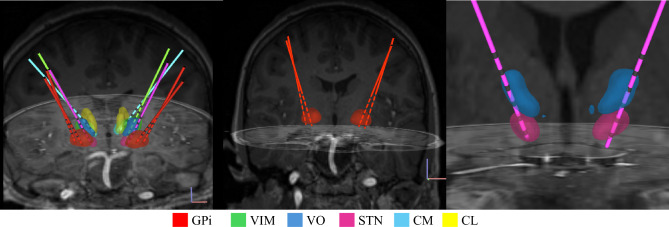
Postoperative localization of implanted DBS leads based on co-registered MRI and CT images for subject S13. The left panel displays all implanted leads and their target regions. For this subject, five leads were implanted in each hemisphere: two in GPi (red), one in VIM (green), one passing through VO (dark blue) and STN (pink), and one spanning CM (light blue) and CL (yellow). The middle panel highlights the GPi region (red), while the right panel focuses on the VO (blue) and STN (pink) regions. These neuroimaging-based localizations guided the selection of recording contacts for EP detection.
